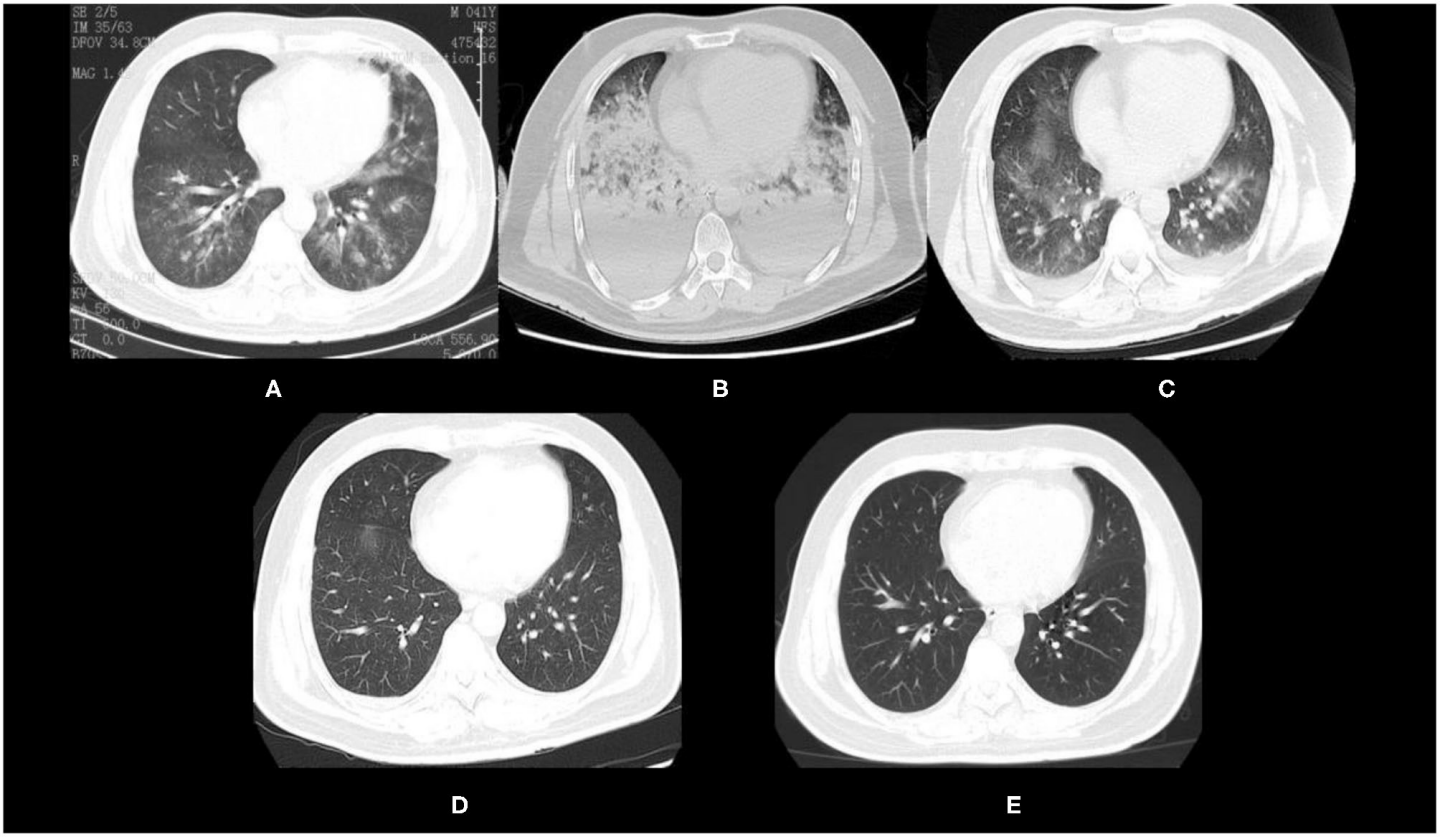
Chest CT of the patient after mixed chemical gas inhalation. (A) Chest CT 2 h after inhalation. (B) Chest CT 20 h after ECMO initiation. (C) Chest CT after ECMO was weaned off. (D) Chest CT 2 days after weaning. (E) Chest CT 54 days after discharge.
